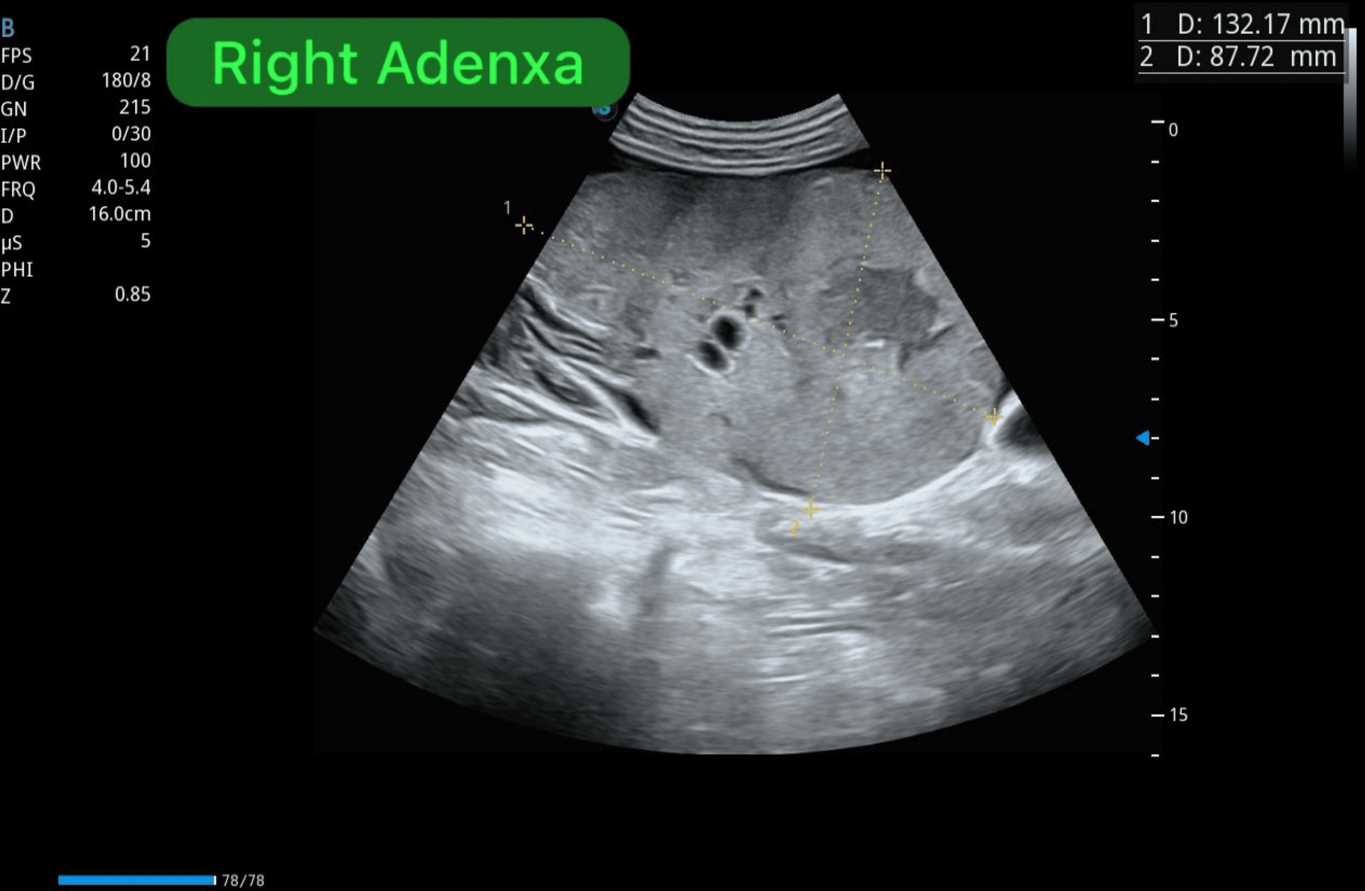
Transabdominal ultrasound of the right adnexa demonstrating a large complex adnexal mass
